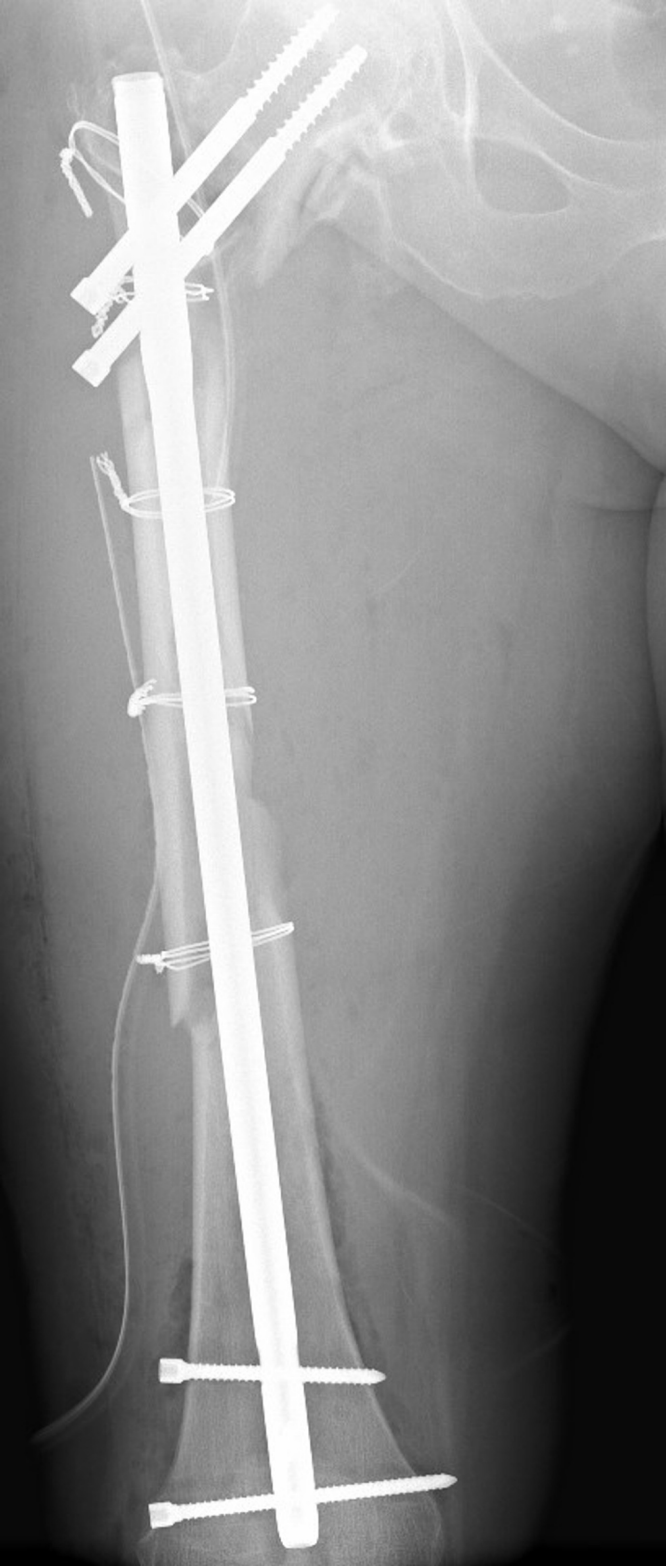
Postoperative X-ray of intramedullary osteosynthesis with a long nail
